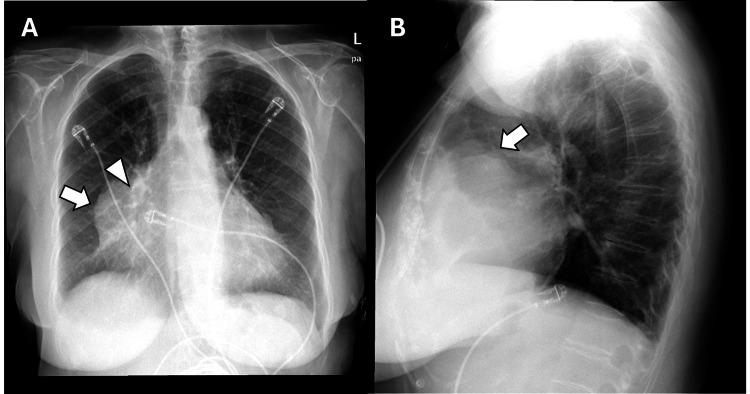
Posteroanterior (A) and lateral (B) chest radiographs. These radiographs show an increased cardiothoracic index secondary to a right paracardiac mass (arrow in A). There is blurring of the right heart contour, and on the contrary, the vessels and bronchi of the right pulmonary hilum can be seen (arrowhead in A), indicating that the mass affects the anterior mediastinum. On the lateral projection, the contour of the mass can be seen superimposed on the cardiac silhouette (arrow in B).
